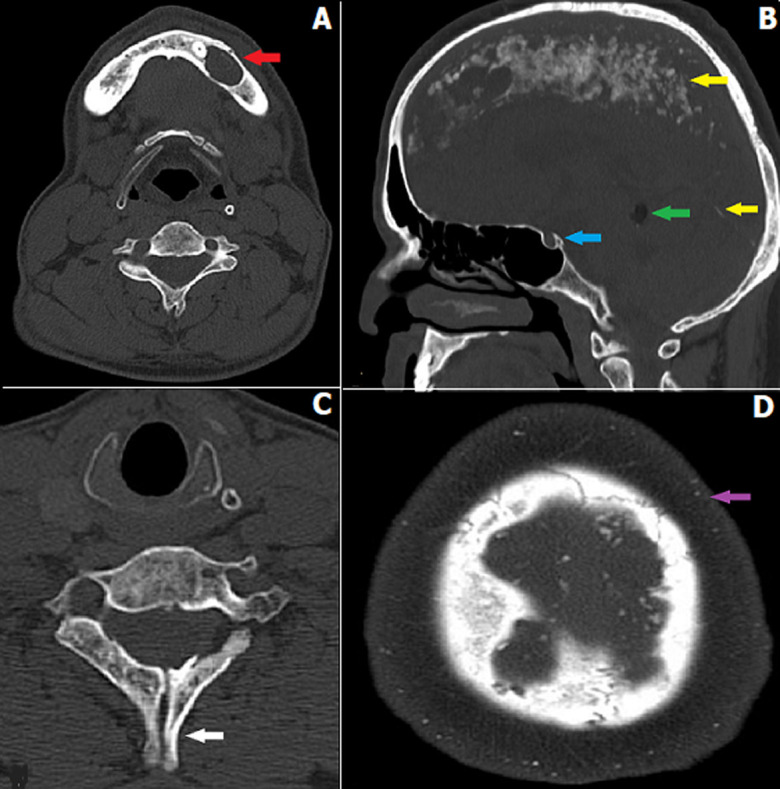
A) computed tomography image showing multiple cystic lesions in the left hemimandible and maxilla consistent with odontogenic keratocysts (red arrow); B) bilamellar falx cerebri and tentorium cerebelli calcifications (yellow arrow), bridging sella (blue arrow), intracranial lipoma (green arrow); C) spina bifida of C6 vertebra (white arrow); D) thickened scalp with multiple calcific foci (purple arrow); diagnosis of Gorlin-Goltz syndrome was made
